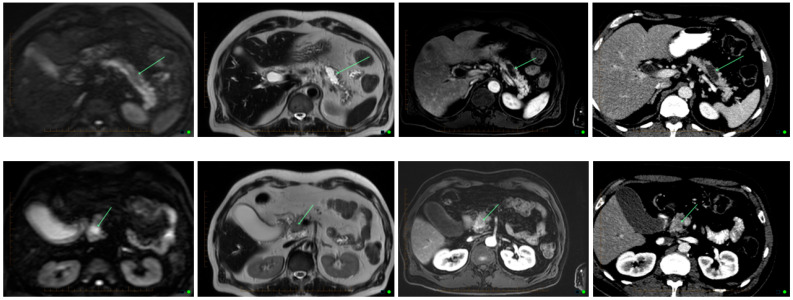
Transformation in IPNM on MRI and CT. From left to right- Diffusion weighted imaging, T2, post contrast T1 and CT images in the same patient. Top row shows a simple cystic lesion consistent with a side branch IPMN, showing no malignant features. Bottom row shows a cystic lesion displaying solid enhancing components with restricted diffusion, consistent with malignant degeneration within an IMPN. Arrows indicate cystic component with no cancer (top), and with cancer (bottom).
